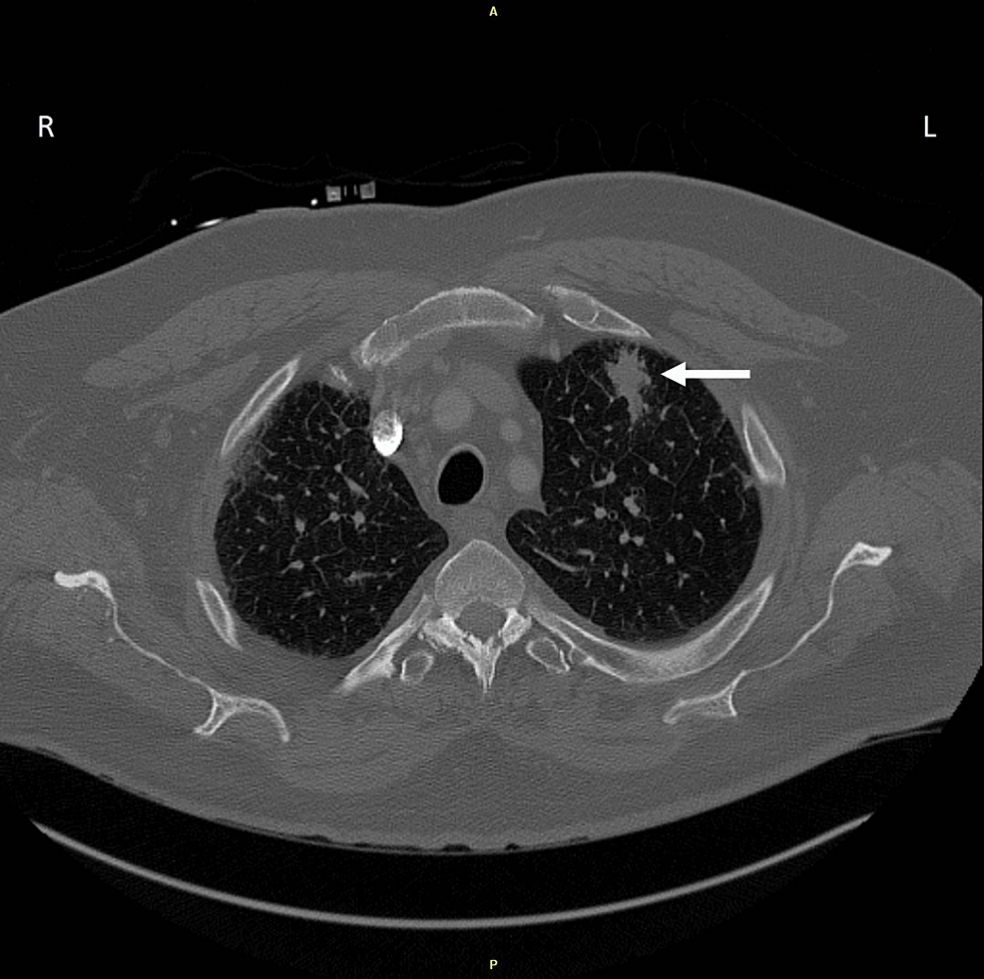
CT chest angiogram with IV contrast on initial presentation demonstrating an 18 x 26 mm solid nodule in the upper left lobe with irregular spiculated margins with concerns for malignancy (arrow) CT: computer topography; IV: intravenous; A: anterior; P: posterior; R: right; L: left
